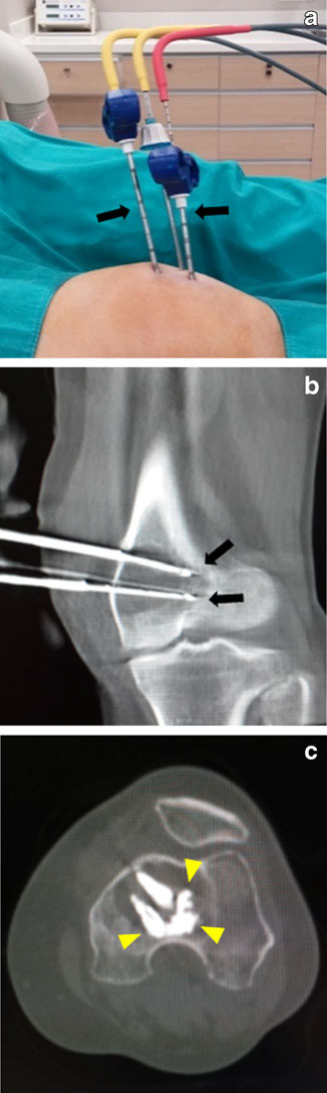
Cryoablation of bone metastasis (the solitary metastasis in the distal femur of the previous patient). a, b First, under local anaesthesia and US guidance, three bone biopsy needles are inserted into the lesion under cone-beam CT guidance, and cryoablation is then performed with needles (*arrows*) inserted coaxially. c After the ablation, the cryoneedles are removed, and cement (*arrowheads*) injection is performed through the outer needles to support the bone
